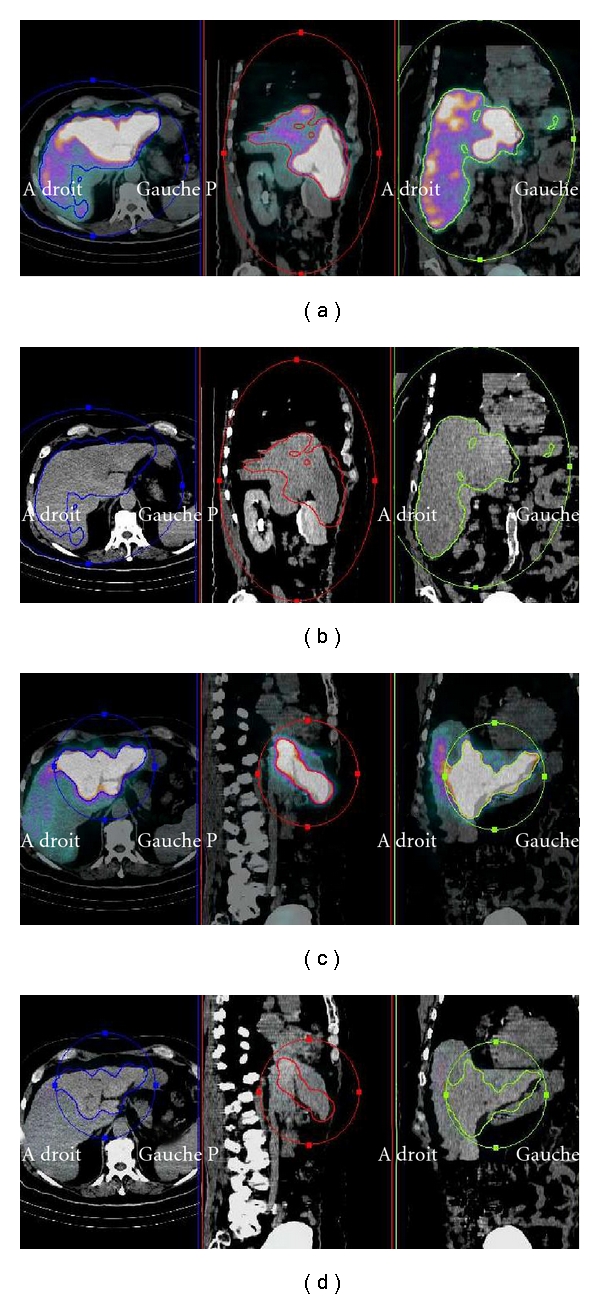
Case report: delineation of the tumoral and injected lover VOI on SPECT/CT whole; injected liver VOI with SPECT/CT color scale (a), whole injected liver VOI with CT scale and visualisation of SPECT isocontour (b), tumoral VOI with SPECT/CT color scale (c) and tumoral VOI with CT scale and visualisation of SPECT isocontour (d).
